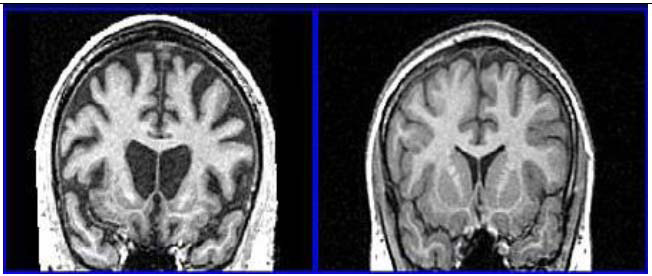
MRI scans of a huntington’s disease (HD) sufferer’s brain (left) and a normal brain (right).
